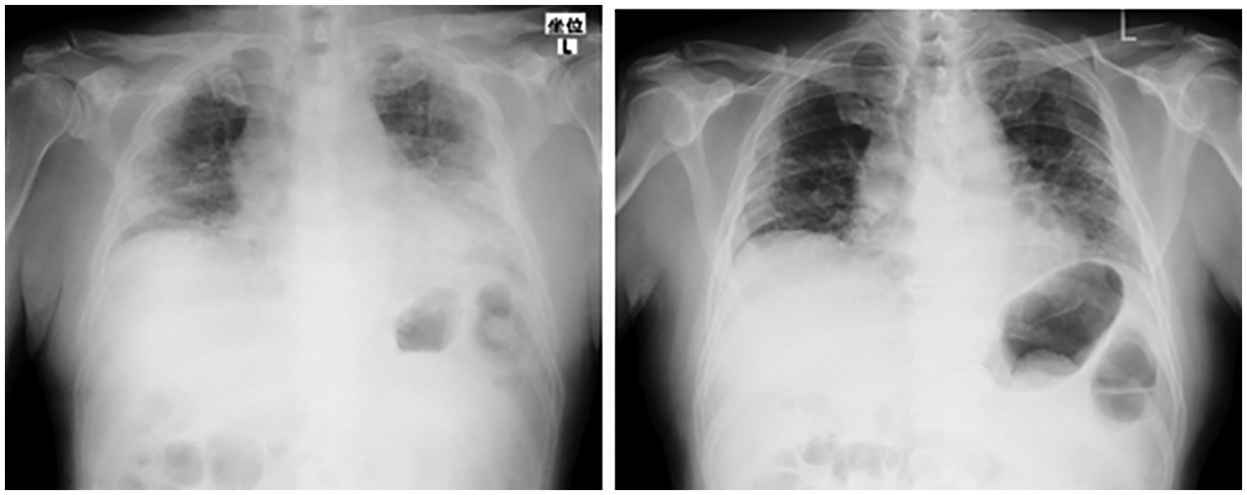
Chest radiograph at the time of onset (case 2). The left side was at the time of admission to our hospital (1 month after onset), and the right was 1 month after admission to our hospital (2 months after onset). Pneumonia was found mainly in the lower lobes on both sides; however, improvement was observed.
